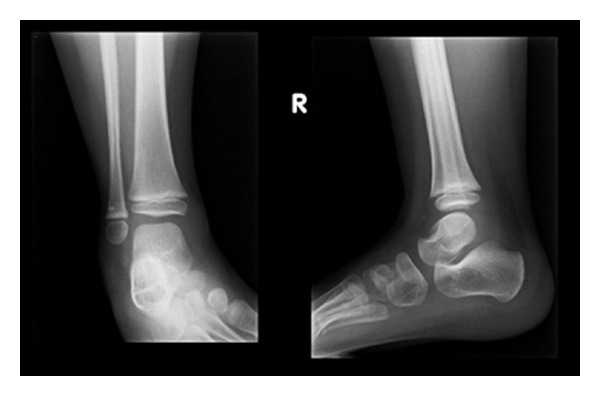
AP and lateral X-ray of the 4-year-old patient with normal findings.
